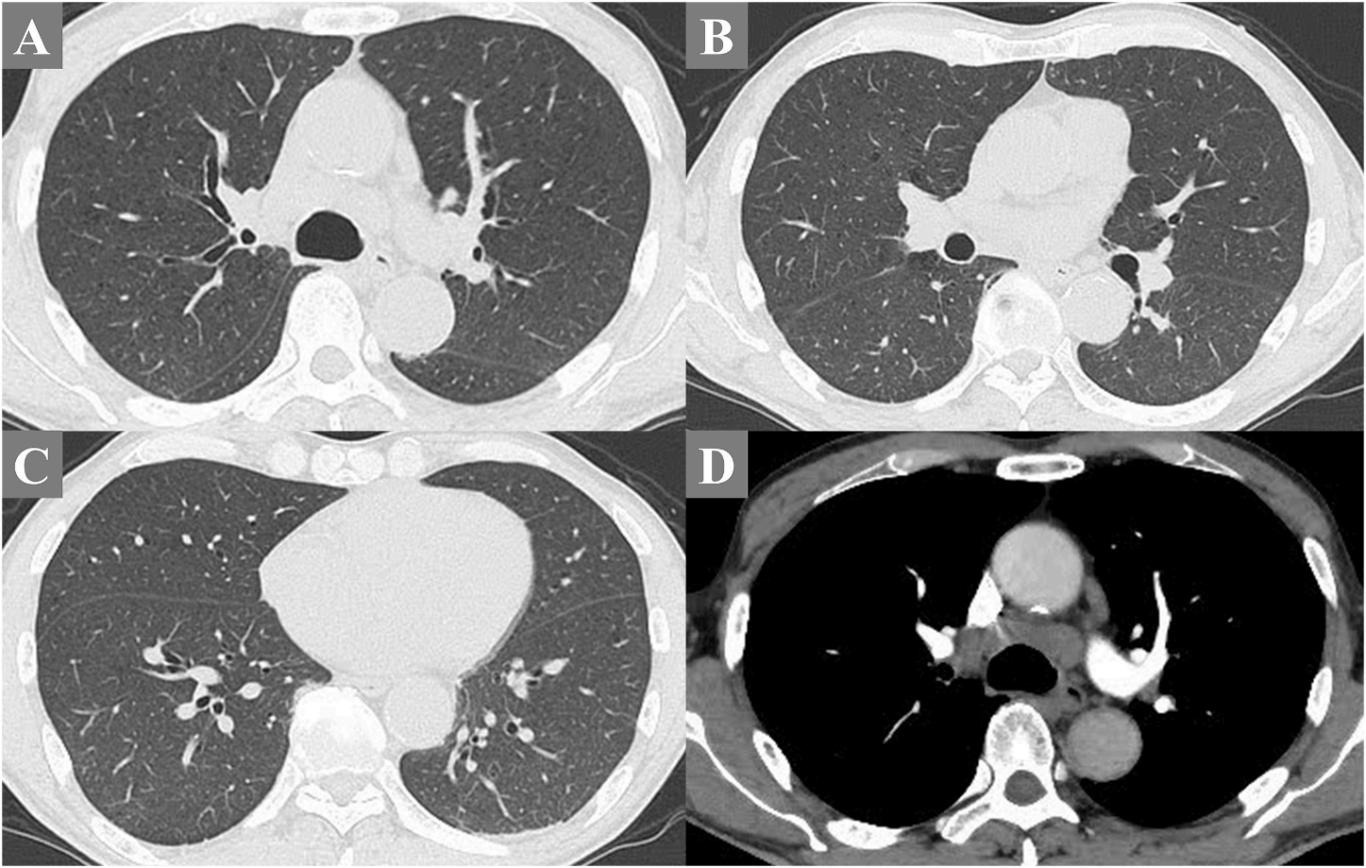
In the plain chest computed tomography (CT), minimal, diffuse increase in pulmonary field density can be observed, with almost normal appearance (A–C). Contrast-enhanced chest CT demonstrates enlargement of the mediastinal hilar lymph nodes (D).
